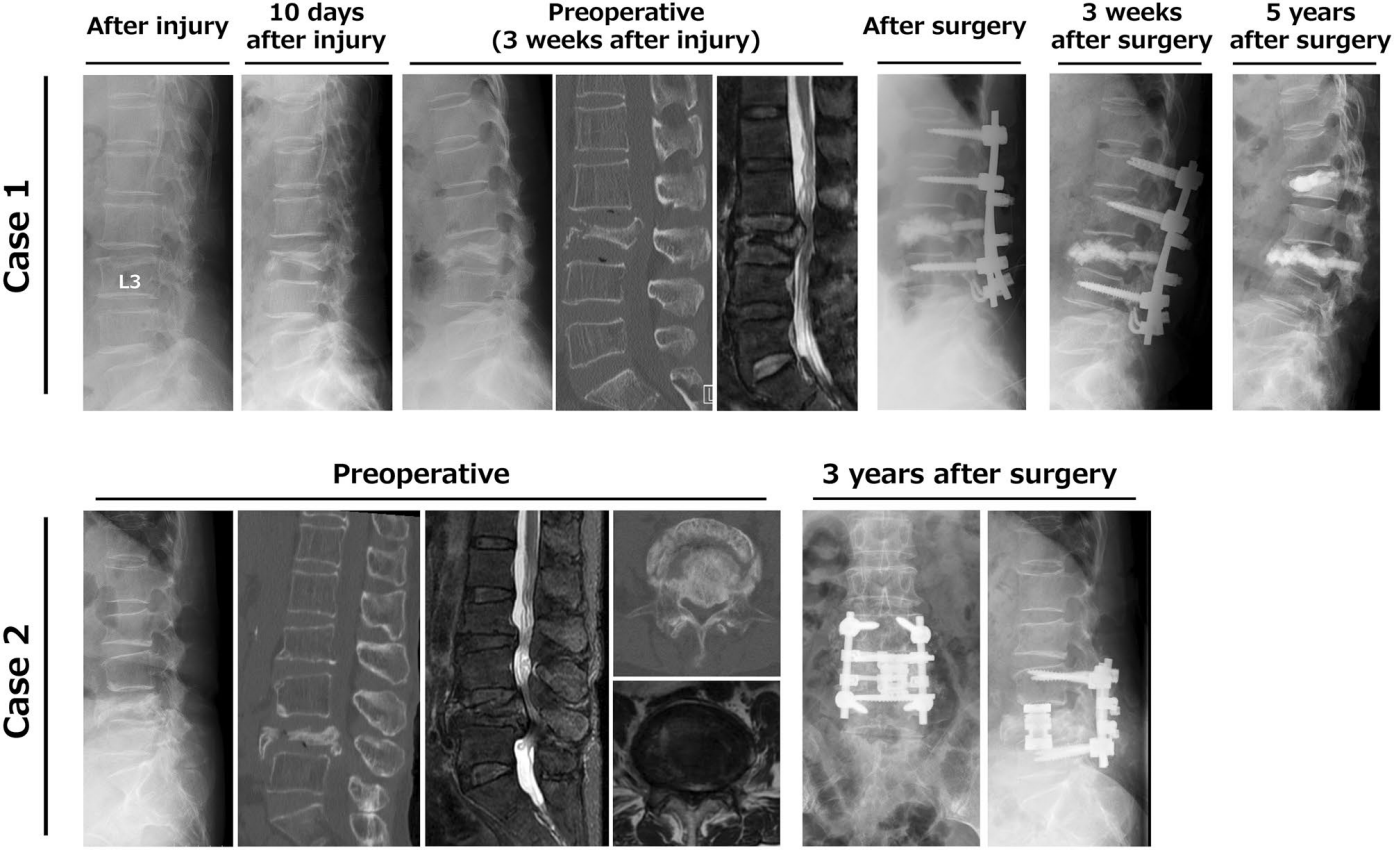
Case 1: A 74-year-old female (Hoehn & Yahr stage III) with severe low back pain and motor dysfunction due to rapidly progressive L3 osteoporotic collapse. Preoperative CT and T2-weighted MRI showed L3 collapse with canal stenosis by bony fragments that had retropulsed into the spinal canal. Instrumentation failure occurred early in the postoperative period with exacerbation of dyskinesia, and required removal of the pedicle screw, but daily activities were maintained despite a decrease in lumbar kyphosis at 5 years after surgery. Case 2: A 70-year-old female (Hoehn & Yahr stage II) with severe low back pain and motor dysfunction due to progressive L4 collapse. Preoperative CT and T2-weighted MRI showed L4 collapse with canal stenosis by bony fragments that had retropulsed into the spinal canal. The patient has been asymptomatic for 3 years after anterior reconstruction and pedicle screw fixation.
